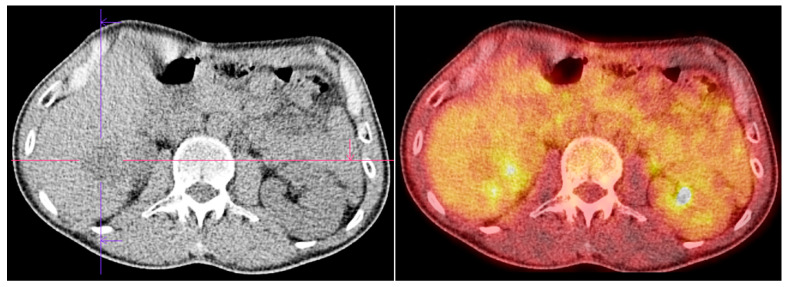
PET/CT shows hypodense liver lesion without significant FDG uptake (SUV max 2.7). There was no abnormal uptake at the esophagogastric anastomosis level, nor in the mediastinal lymph nodes. However, the scan showed a metabolically active, FDG-avid lytic lesion in the right sacral bone, measuring 55 × 65 mm (SUVmax 11.4).
